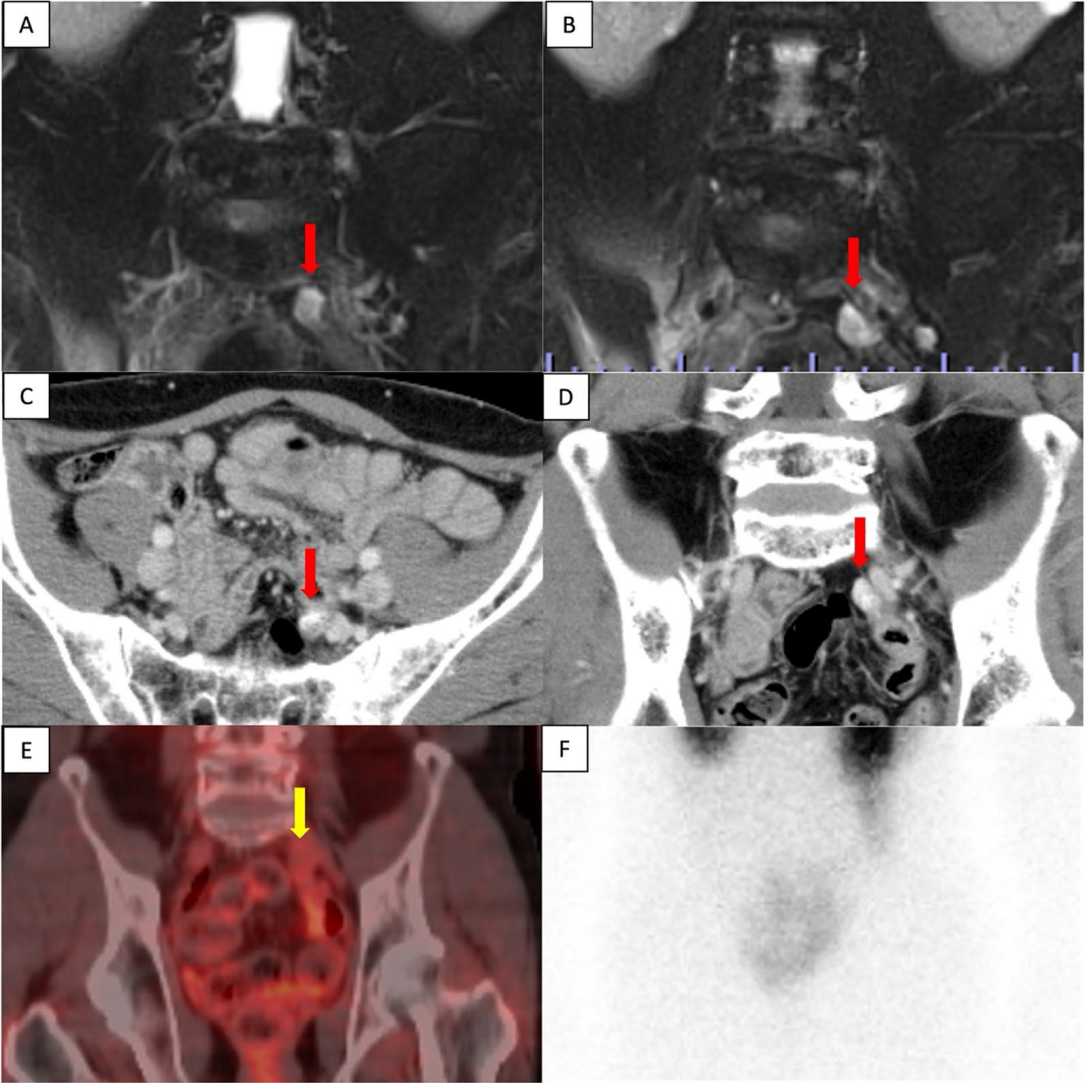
A nodular lesion in the pelvis (arrow). A, B A contrast-enhanced MRI (T1-weighted with fat suppression) showed an intrapelvic lesion increasing in size from 13 to 19 mm after 4 months. C, D A contrast-enhanced CT showed a 20-mm nodular lesion with a contrast effect. E FDG-PET/CT showed a low accumulation of FDG in the nodular lesion. F SRS showed the low expression of somatostatin receptors in the nodular lesion. *CT *computed tomography, *MRI* magnetic resonance imaging, *SRS* somatostatin receptor scintigraphy, *PET* positron-emission tomography, *FDG* fluorodeoxyglucose
